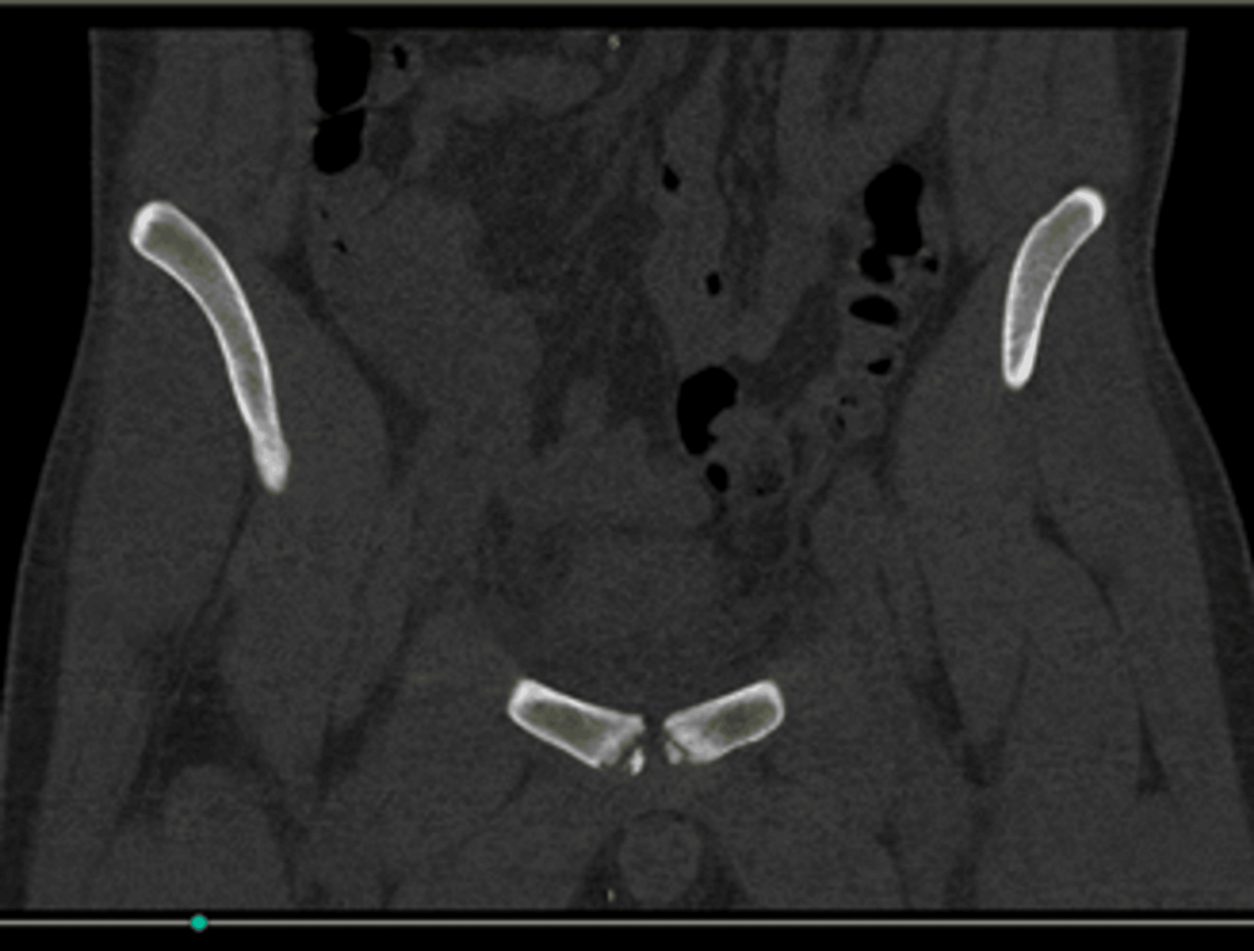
CT scan showing evidence of osteitits pubis Foci were insufficient in size to allow image-guided drainage.
